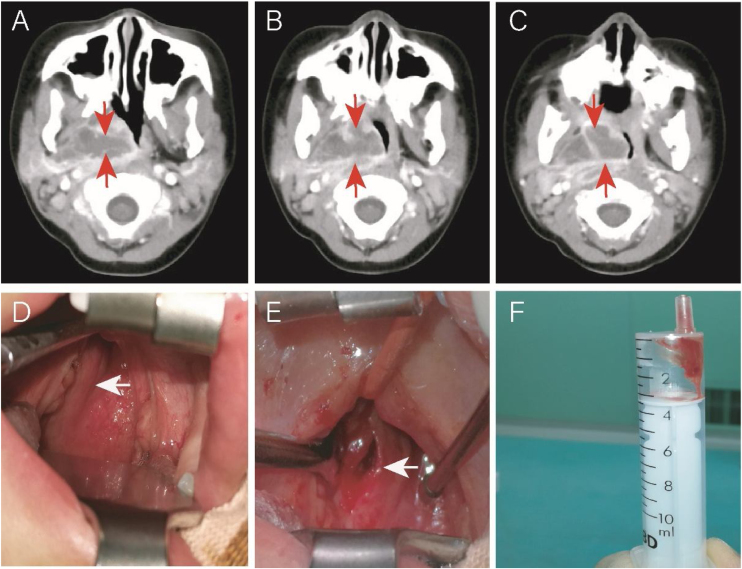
Case 2: a patient with KD and deep neck abscess who underwent incision and drainage. (A, B, and C) Enhanced neck computed tomography showing a neck abscess. (D) Right parapharyngeal and retropharyngeal eminence (arrowhead). (E) After incision and drainage (arrowhead). (F) One milliliter of serous fluid was drained without bacterial growth in cultures.
